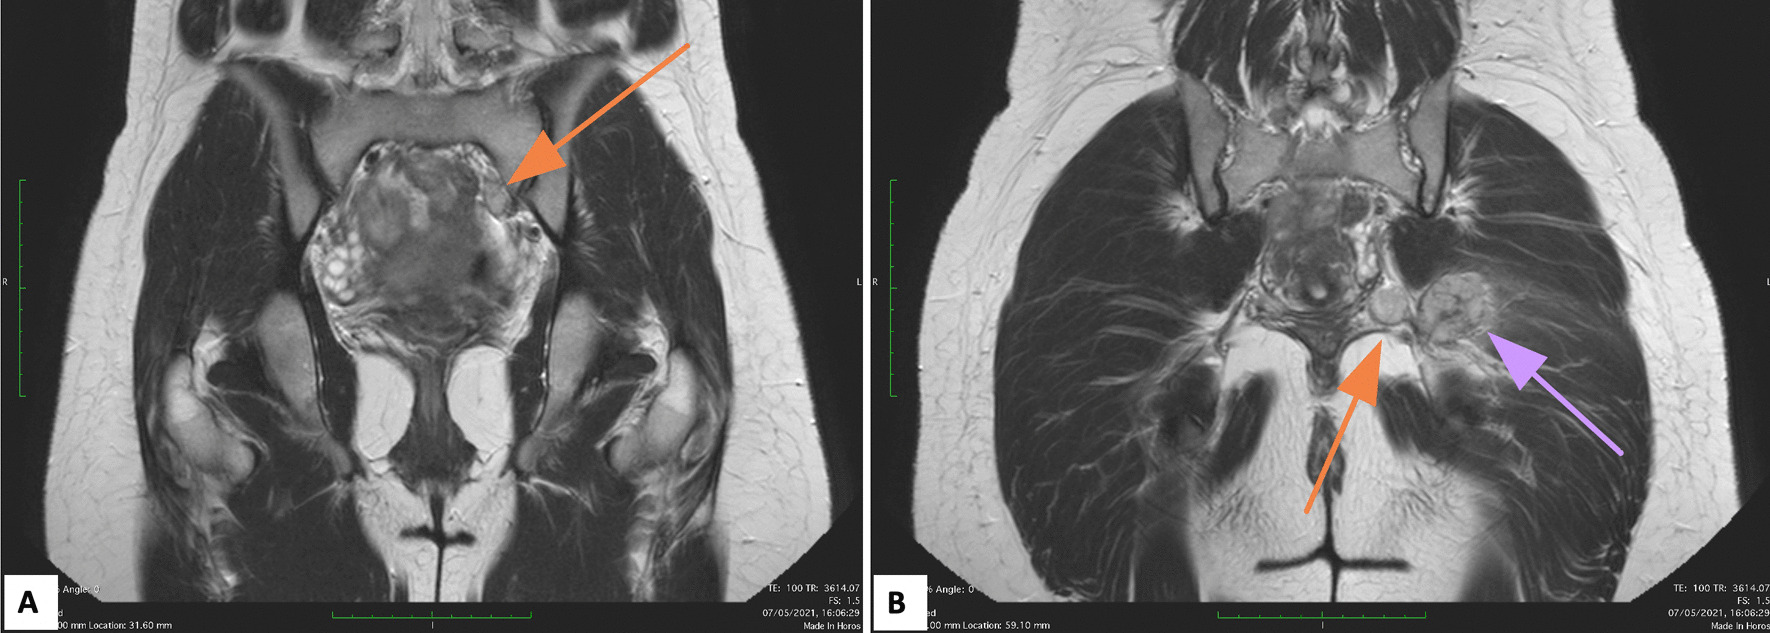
Magnetic Resonance Imaging (MRI) coronal T1 weighted sequencies of the pelvis: A nodule (15 mm) at the left external iliac level (orange arrow); B gluteal nodule (purple arrow) and two contiguous nodules in the ischiorectal fossa (orange arrow)
